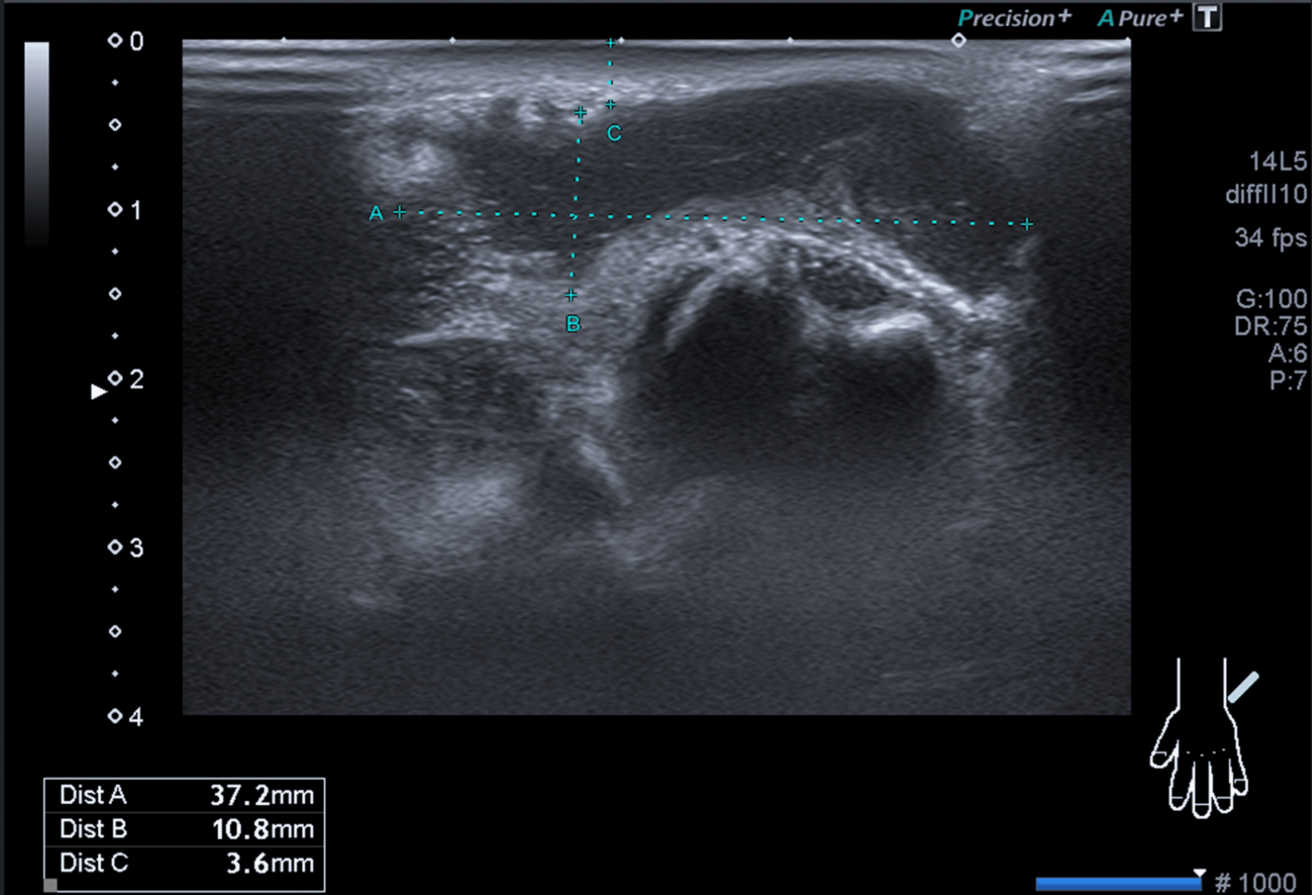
Extensor‑compartment abscess on longitudinal ultrasound Longitudinal musculoskeletal ultrasound of the dorsal left wrist showing an anechoic collection with internal echoes and septations along the extensor tendon sheath. Measurements are displayed as Dist. A (37.2 mm transverse width), Dist. B (10.8 mm anteroposterior depth), and Dist. C (3.6 mm from the skin surface). These findings confirmed the diagnosis of tenosynovitis with abscess formation and guided the surgical approach for open drainage.
